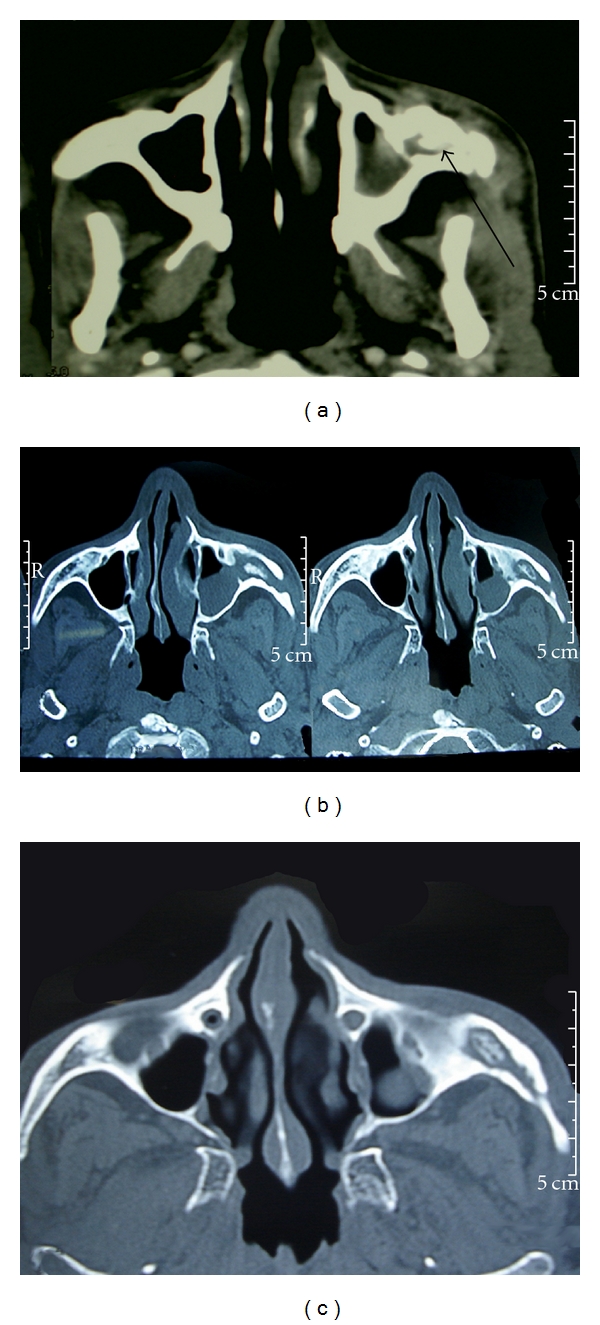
CT scan showing osteomyelitis of left maxilla and zygoma.
